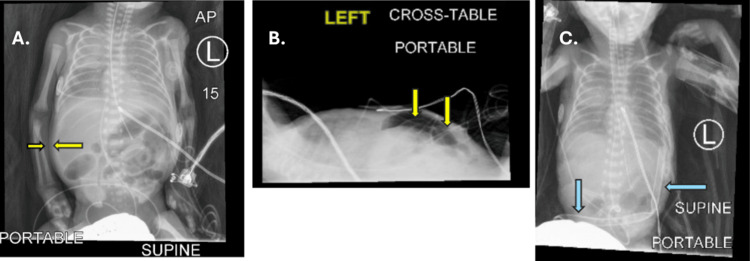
Radiographic findings of spontaneous intestinal perforation in an extremely premature neonate A. Supine abdominal radiograph demonstrating lucency concerning for free intraperitoneal air (yellow arrows). B. Cross-table lateral radiograph confirming pneumoperitoneum beneath the anterior abdominal wall (yellow arrows). C. Follow-up supine radiograph status post Penrose drain placement (blue arrows).
